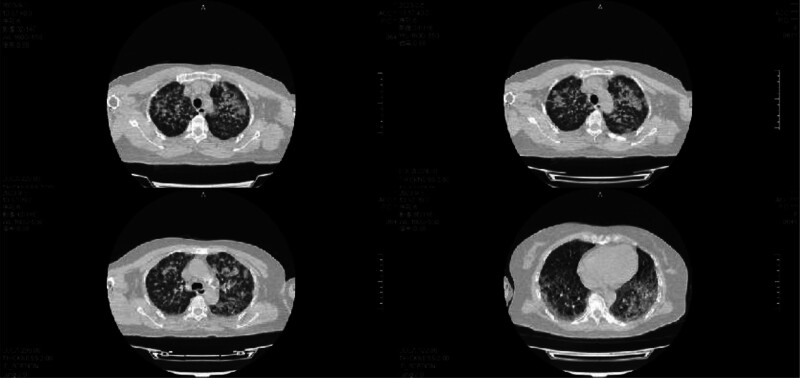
Chest CT on September 5, 2023, showed extensive bilateral patchy and flocculent high-density opacities. CT = computed tomography.
